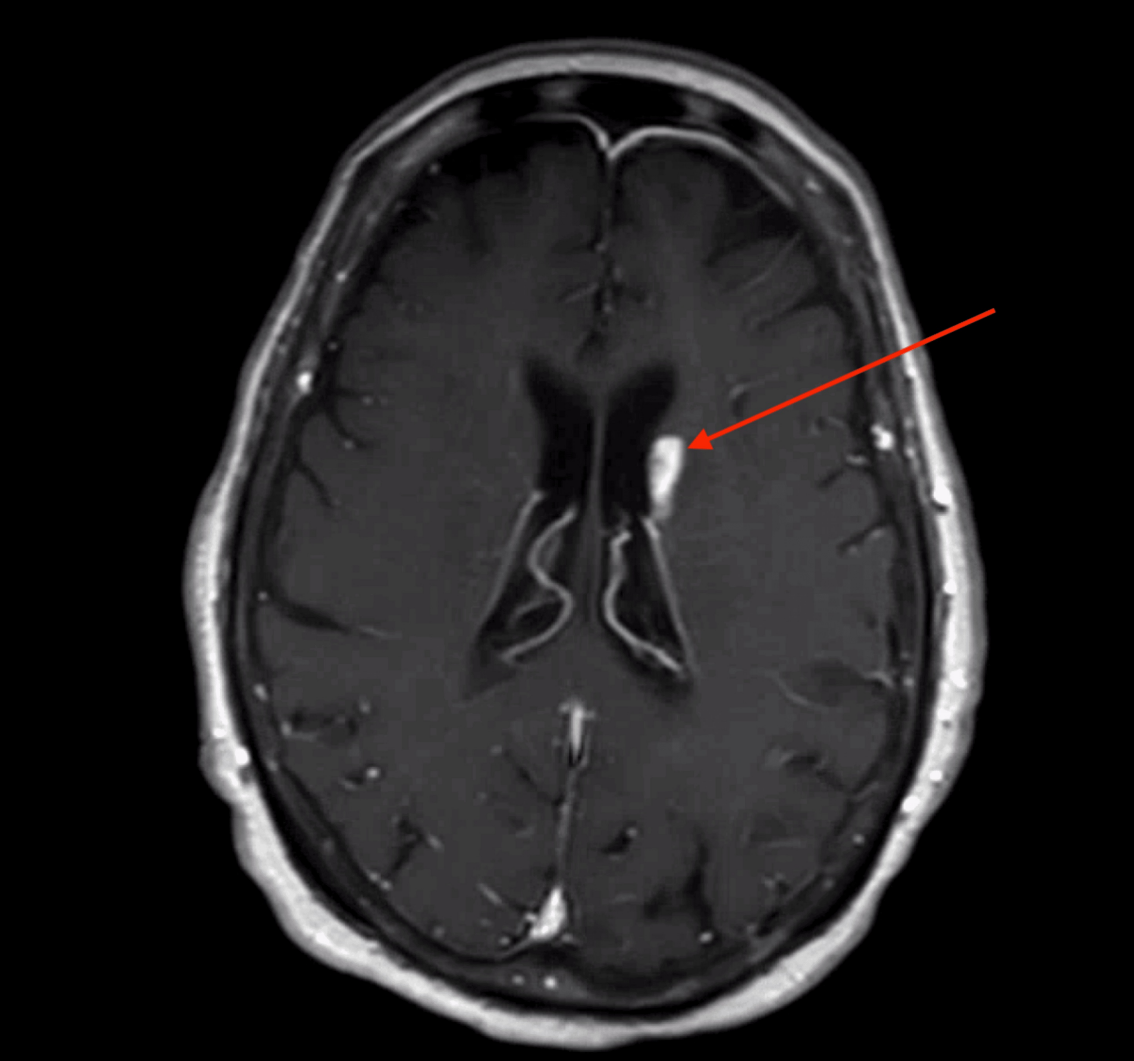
MRI of the brain with contrast (axial T1 view) showing findings concerning for septic emboli on hospital day 11. Largest lesion at the left lateral ventricle measuring 1.6 × .6 cm (red arrow).
